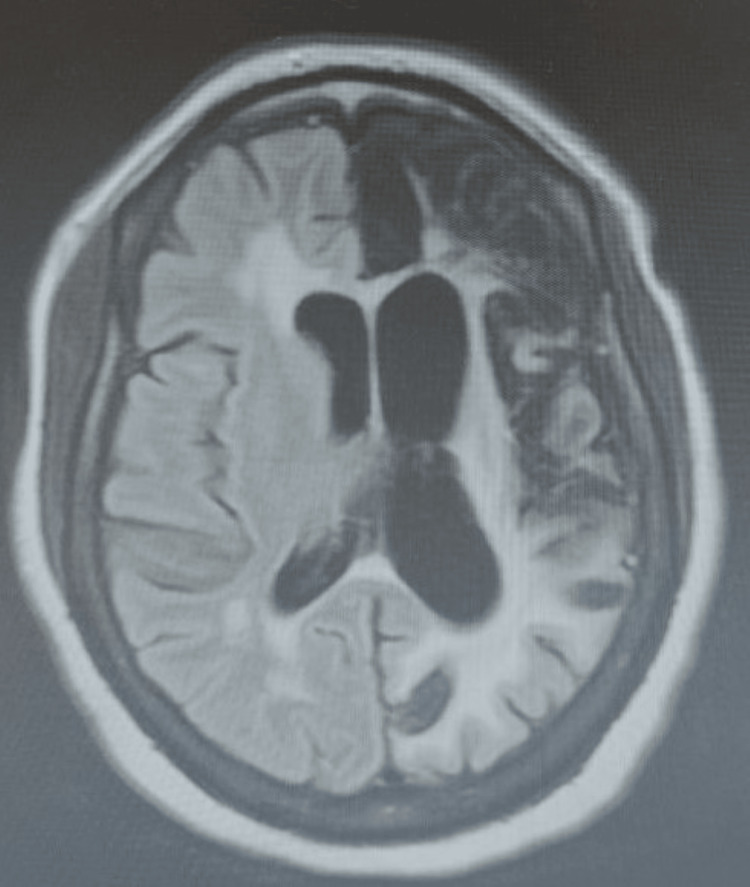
MRI FLAIR head demonstrates a large area of infarction in the left cerebral hemisphere with extensive white matter gliosis and associated ex vacuo dilatation of the lateral ventricle. MRI FLAIR: magnetic resonance imaging with fluid-attenuated inversion recovery
